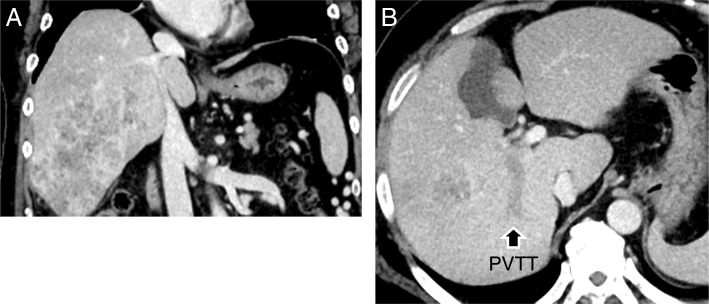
Dynamic CT scan on admission to our hospital. a Coronal view (portal phase). b Axial view (portal phase). Dynamic CT showed a large diffuse-type HCC with a PVTT in the right posterior branch of the portal vein (arrow)
